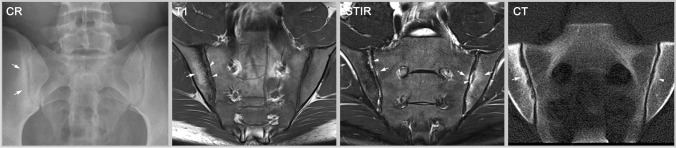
Sacroiliitis—findings typical for axSpA. The radiography (CR) shows sclerosis and erosions (arrows) in the right SIJ whereas the left SIJ appears normal. T1w-MRI additionally reveals fatty marrow metaplasia (arrow) and erosion (arrowheads) in the right SIJ; and STIR displays inflammatory bone marrow lesions (arrows) bilaterally, especially in the left SIJ, as well as inflammation within the joint space (arrowheads) bilaterally. Computed tomography (CT) depicts erosions and sclerosis with high resolution (arrow) but cannot detect active bone marrow inflammation. In the left SIJ, CT shows only a slight erosion of the iliac cortical surface and mild sclerosis (arrowhead). All three modalities are typical for sacroiliitis caused by axial spondyloarthritis (axSpA)
